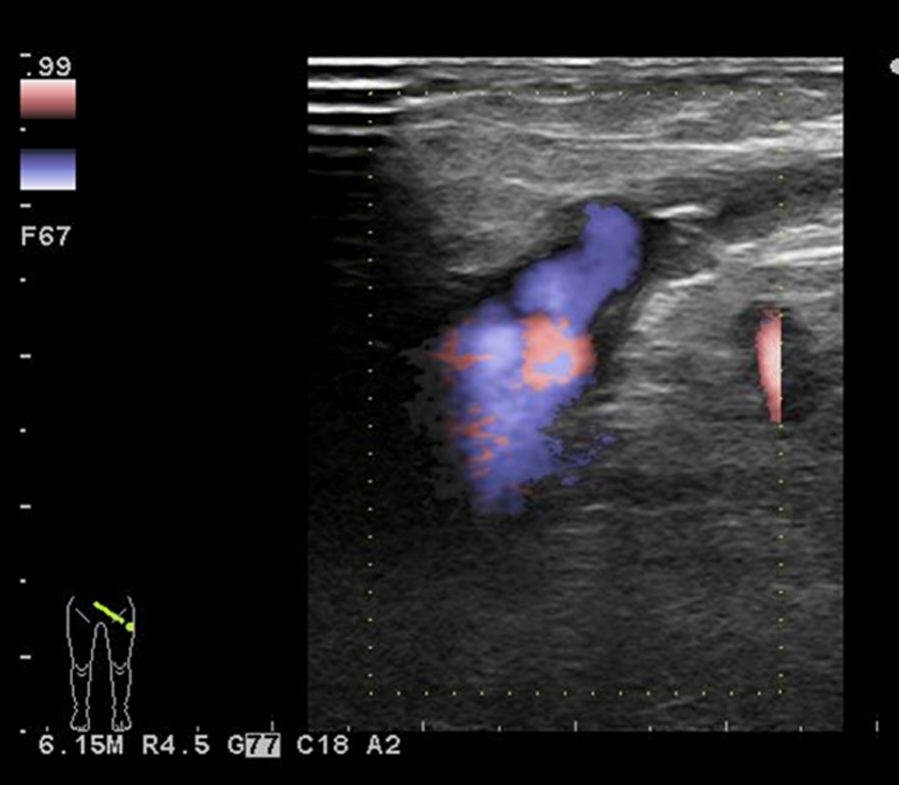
Follow-up duplex sonography revealed that the success of EVLA of the left GSV and the patency of the deep vein system
